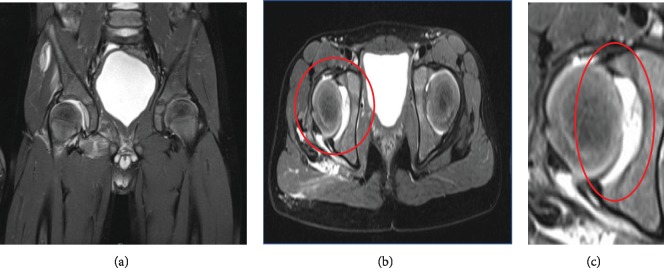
(a–c) Axial and coronal pd_tse_fs MRI pictures showing a type 1A lesion (Czerny classification) of the anterior-superior acetabular labrum and ruptured ligament of the femoral head.
